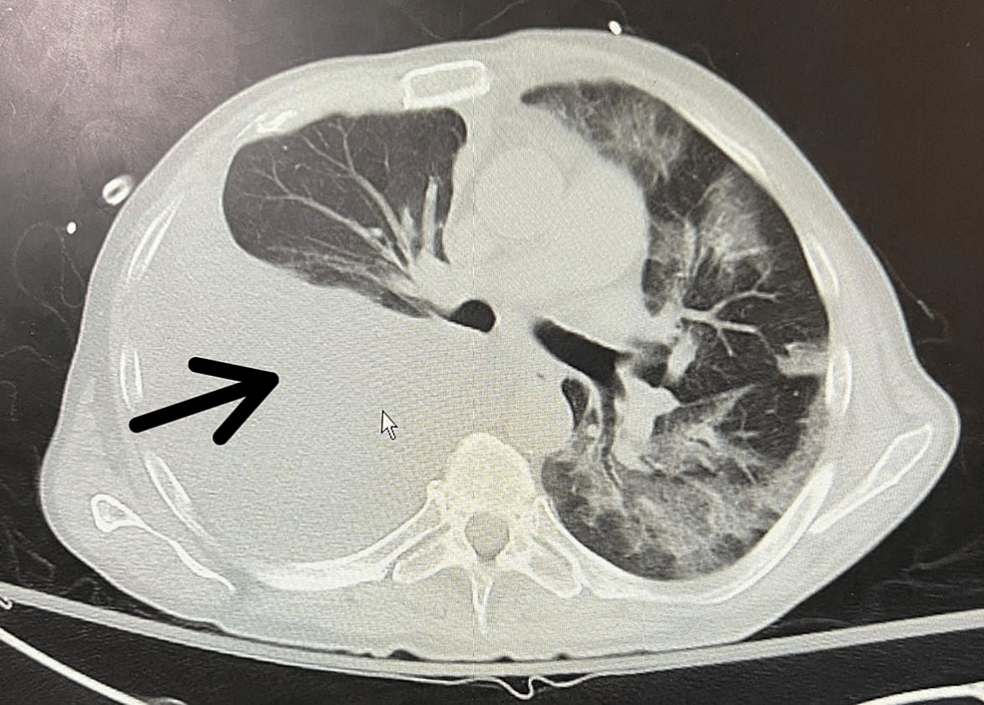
Chest CT scan without contrast showing a large right-sided pleural effusion leading to complete collapse of right middle and lower lobes. CT: computed tomography
